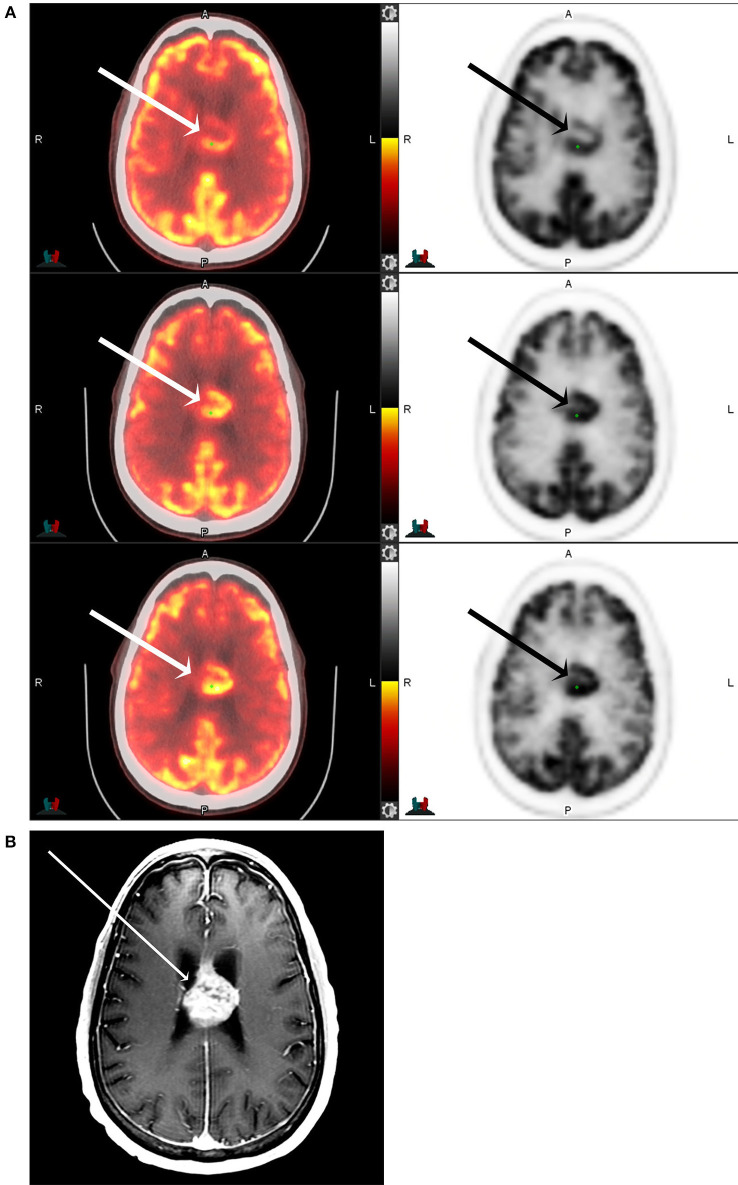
F18 FDG PET CT (A) and T1 postcontrast MRI of the brain (B) of a 71-year-old male with IDH-mutant, glioblastoma involving the corpus callosum and cingulate gyrus (arrows) at 1-, 5-, and 8-h following F18-FDG injection. The maximum tumor SUV, as well as the tumor to background ratios, are seen to increase with increasing time from the injection.
